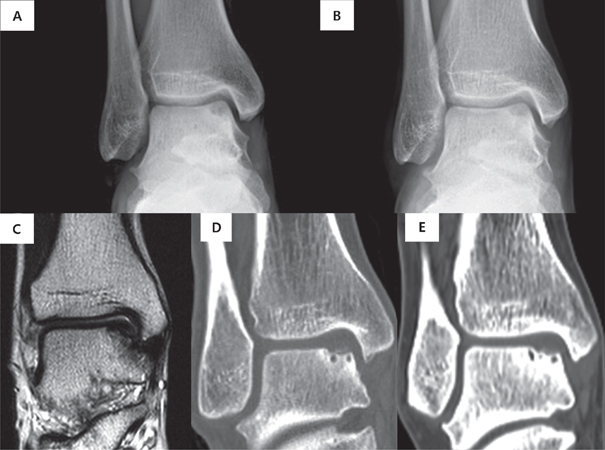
A 29-year-old man with OCL. (A) Initial standing radiograph and (C) initial MRI showed medial OCL. (D) CT at 1-year follow-up, (B) standing radiograph and (E) CT at 9-year follow-up showed no change in lesion size. The AOFAS ankle–hindfoot score improved from 92 to 100.
